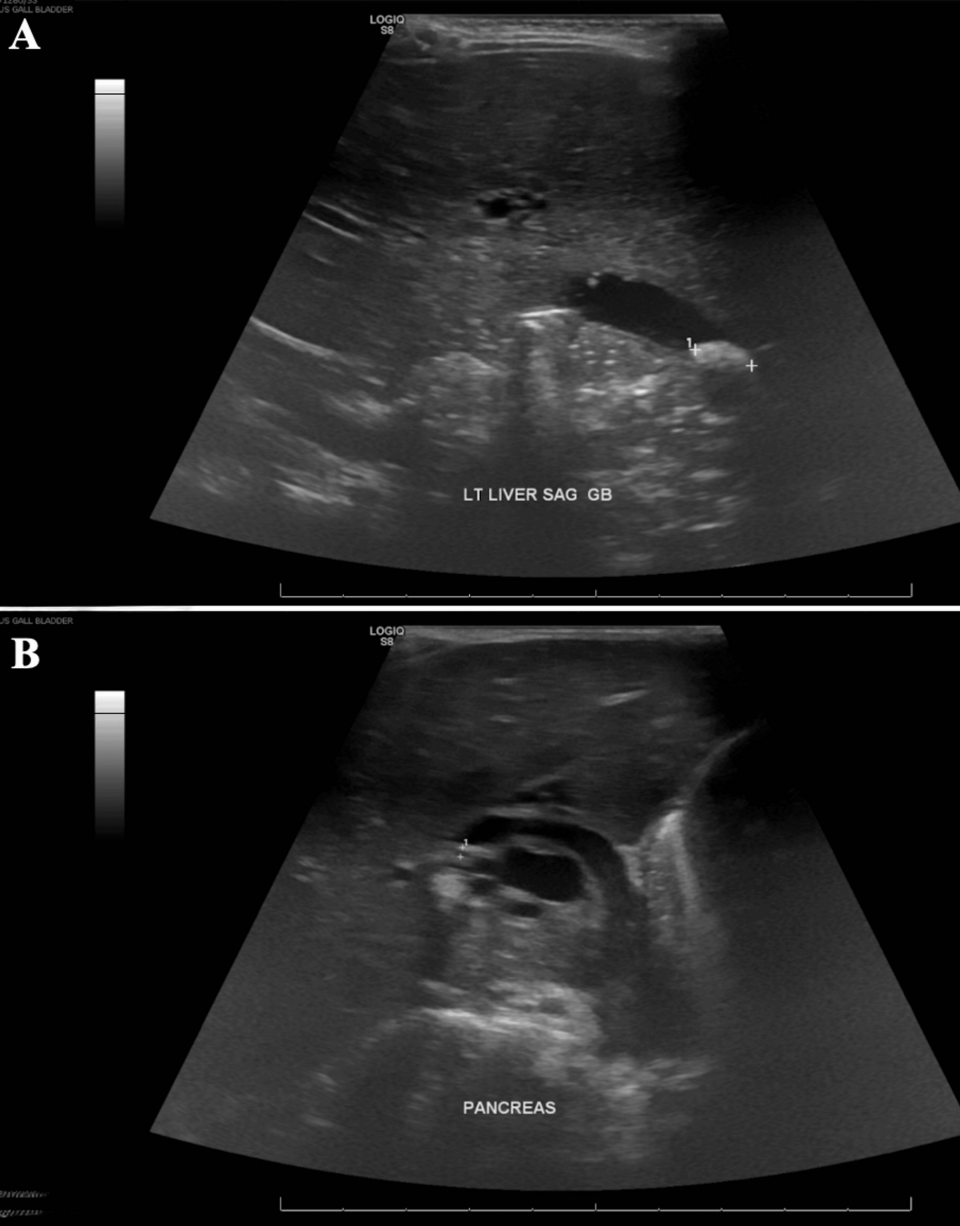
Repeat abdominal ultrasound Repeat abdominal ultrasound (A: liver sagittal view, B: pancreas view) showing the intrahepatic ducts and CBD remaining dilated with multiple gallstones, the largest of which measuring 9 mm. No gallbladder wall thickening. CBD: common bile duct
